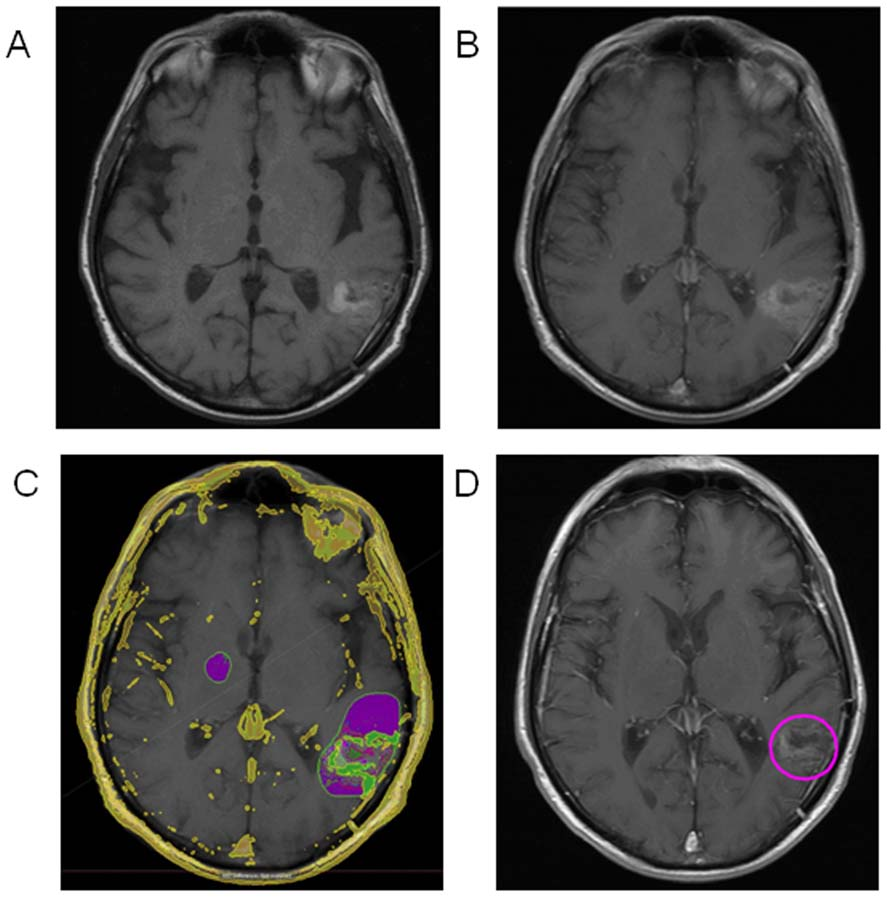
Detection of Enhancement that is Obscured by Blood Products. A) Uncontrasted T1-weighted axial image showing resection cavity blood products (bright on T1). B) T1-weighted post-contrast axial image showing the difficulty in determining residual enhancing tumor. C) Our volumetric analysis is able to detect the obscured enhancing tumor tissue (shown in green). D) T1-weighted post-contrast axial image at 2.5 months later after the blood has resolved verifying the underlying enhancing tumor volume.
